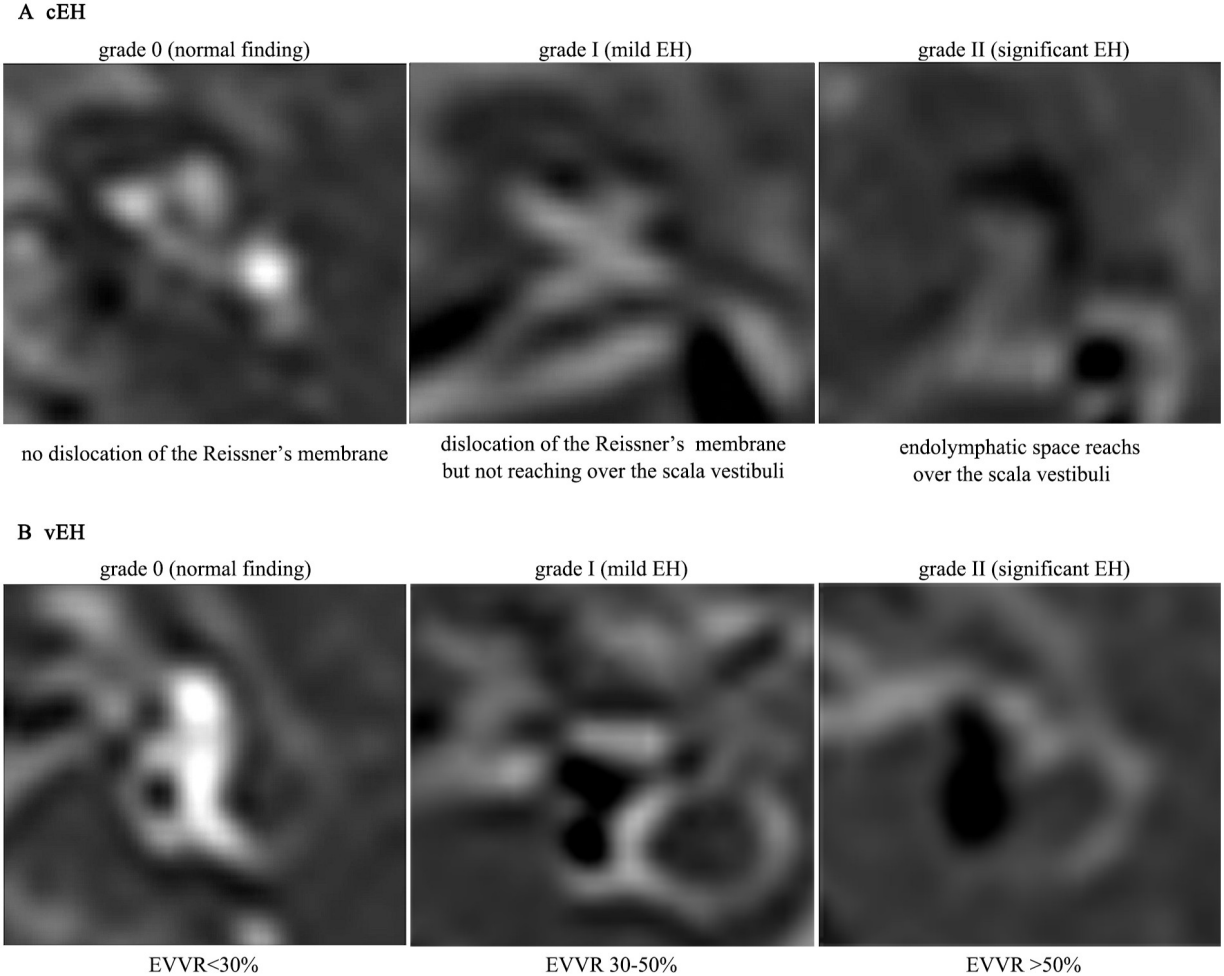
Criteria for EH-grading of the cochlea and vestibule in MRI performed 4 h after intravenous and 24 h after intratympanic administration of Gd-based contrast media (IV-Gd + IT-Gd MRI). (A) The criterion for cEH was the dislocation of the Reissner’s membrane. (B) The decisive factor for vEH is the EVVR. EH, endolymphatic hydrops; MRI, magnetic resonance imaging; Gd, gadolinium; IV-Gd, intravenous injection of gadopentetate dimeglumine; IT-Gd, intratympanic injection of gadopentetate dimeglumine; cEH, cochlear EH; vEH, vestibular EH; EVVR, endolymph to vestibule-volume.
